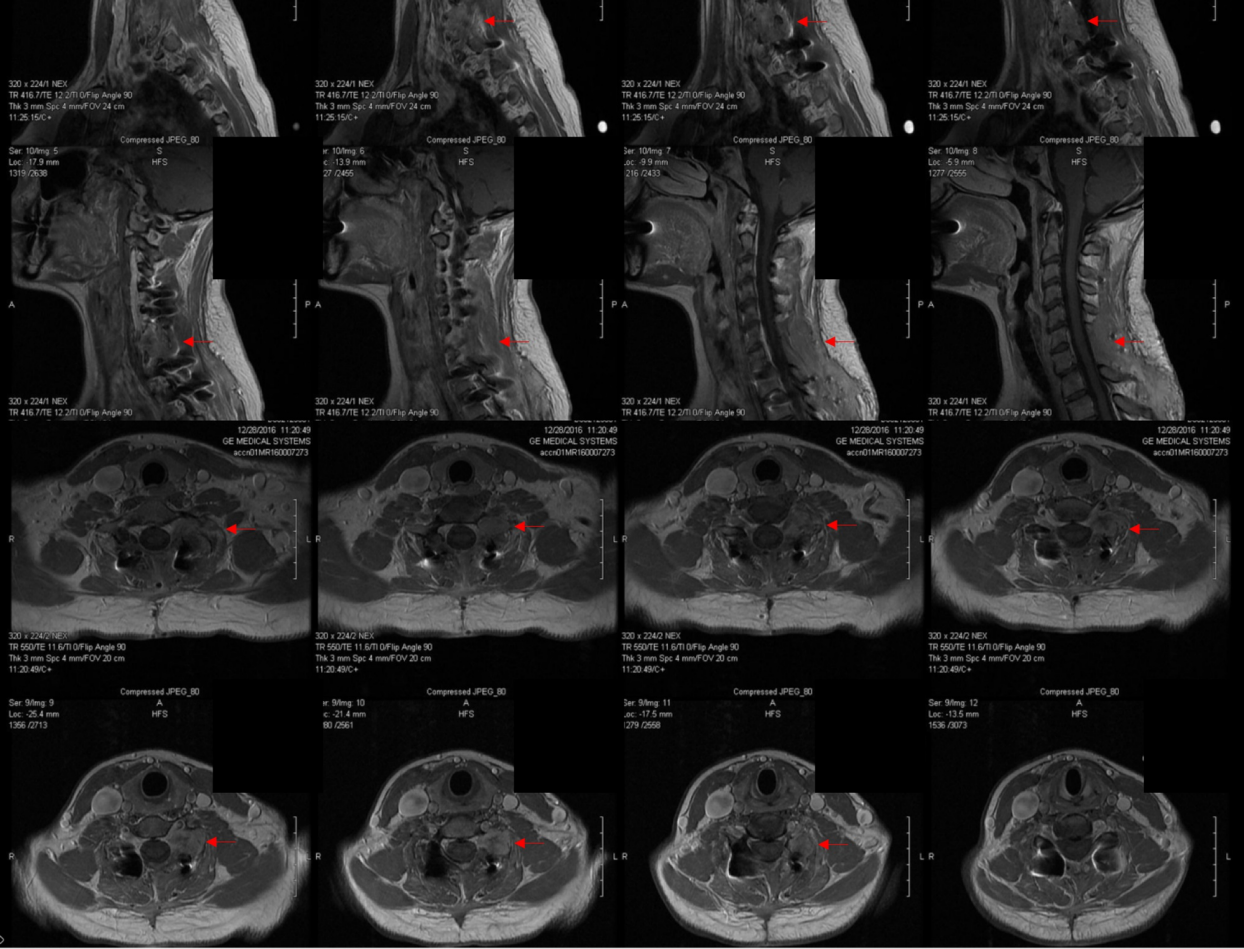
Postoperative MRI after the initial surgery Postoperative contrasted sagittal and axial MRI after the initial surgery shows a subtotal resection with residual tumor (red arrows) at the facet and near the left vertebral artery MRI: magnetic resonance imaging
